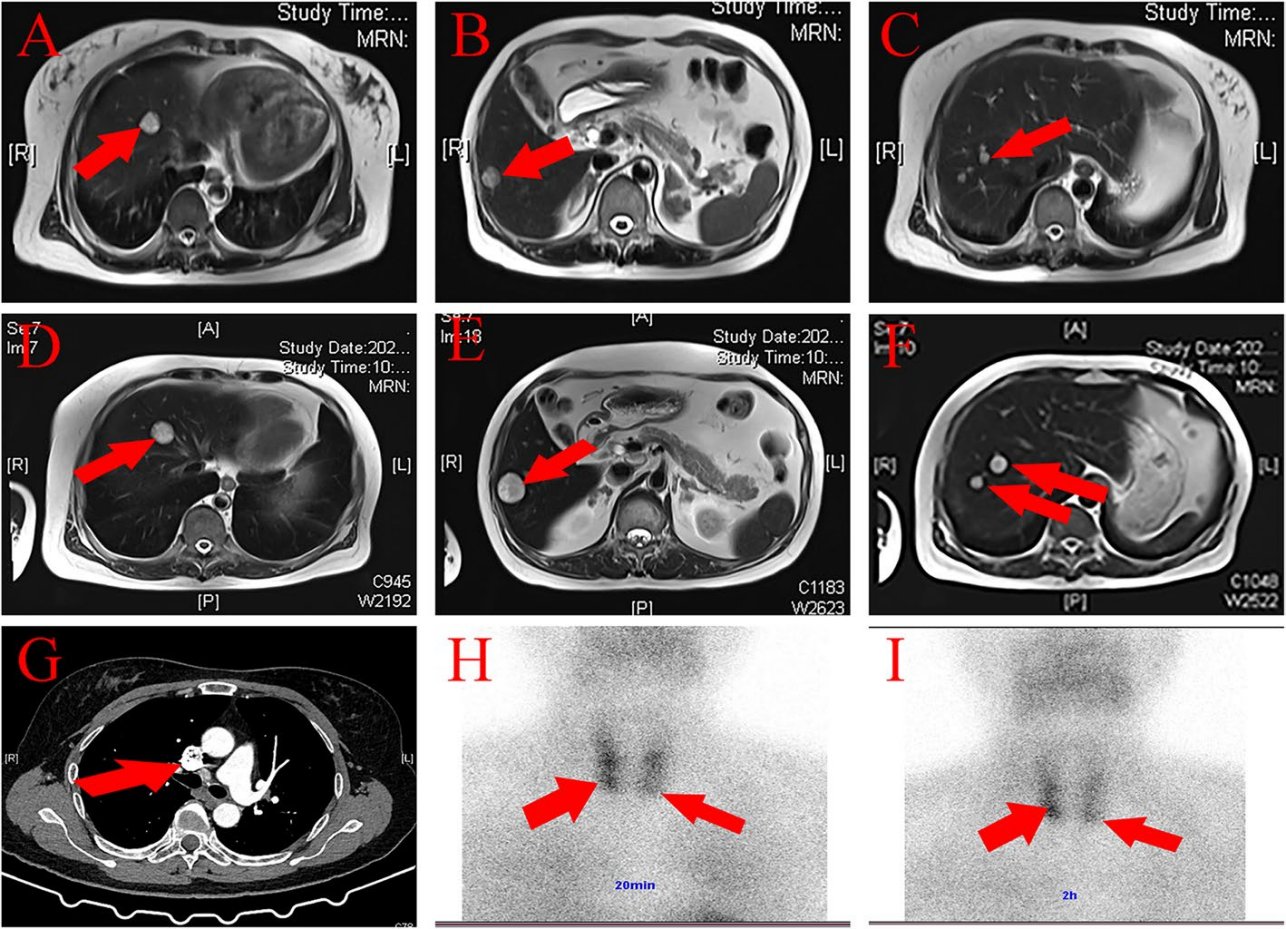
Clinical features before hepatectomy. The red arrows point to the location of lesions. A–C Multiple lesions were found in VI, VI, and VII segments with the largest diameter of about 1.8 cm by T2-weighted magnetic resonance image of liver in July 2020. D–F Multiple lesions in VI, VI, and VII segments were larger than before, with a largest diameter of about 2.9 cm in December, 2020. G In July 2020, computed tomography (CT) enhanced arterial phase showed the tumor, about 3.7 cm × 2.7 cm in size, located in the middle lobe of right lung and exhibited a inhomogeneous enhancement. H, I In December, 2020, Technetium-99m SESTAMIBI at 20 min and 2 h suggested parathyroid adenomas located in the lower poles of both sides
